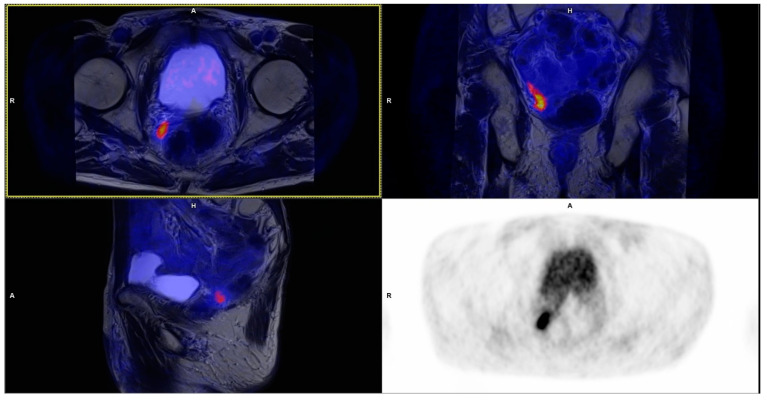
Local recurrence detected in patient with serum PSA level 0.22 ng/mL. The lesion is localized directly to the rectal wall. T2_tse_bl MR images fused with PET (upper images and lower left) and PET AC images (lower right).
